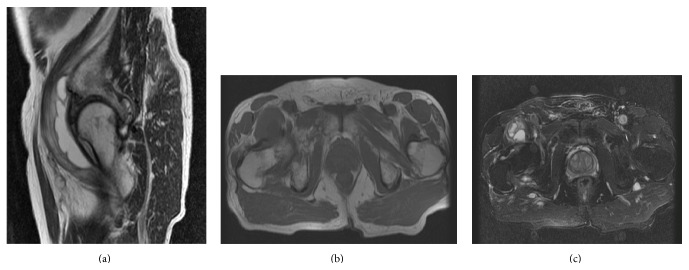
Magnetic resonance imaging (MRI). An elongated neoformation with predominantly fluid content is shown, multiloculated and localized in the anterior-lower aspect of the right hip joint. (a) Sagittal T2-weighted scan. (b) Axial T1-weighted magnetic resonance (MR) image. (c) Axial short tau inversion recovery (STIR) MR image.
